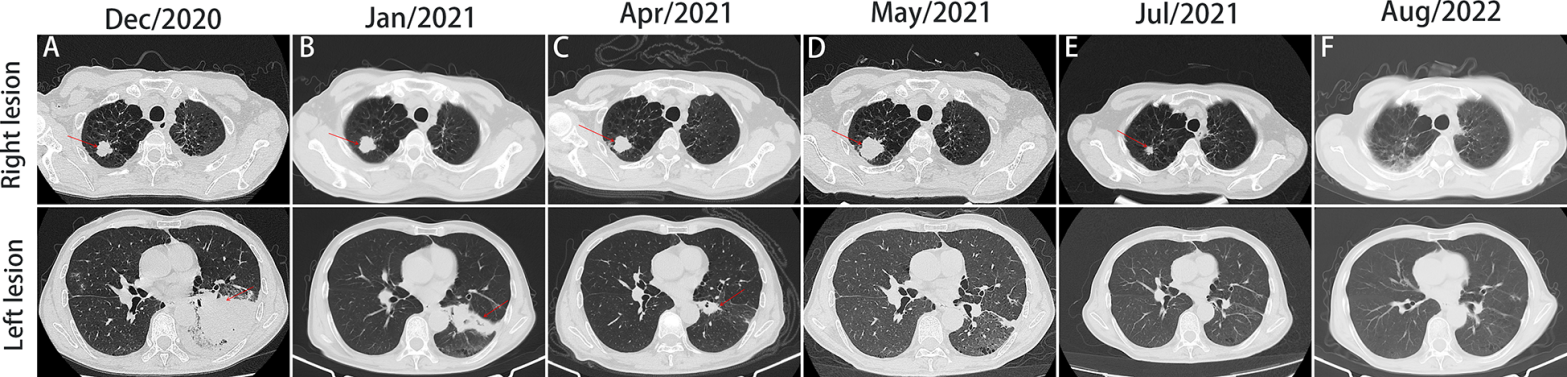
Computed tomography (CT) scan of the patient throughout the whole course of diagnosis and treatment. Figures A-E, imaging changes of chest CT (mediastinal window and pulmonary window) at different times.
